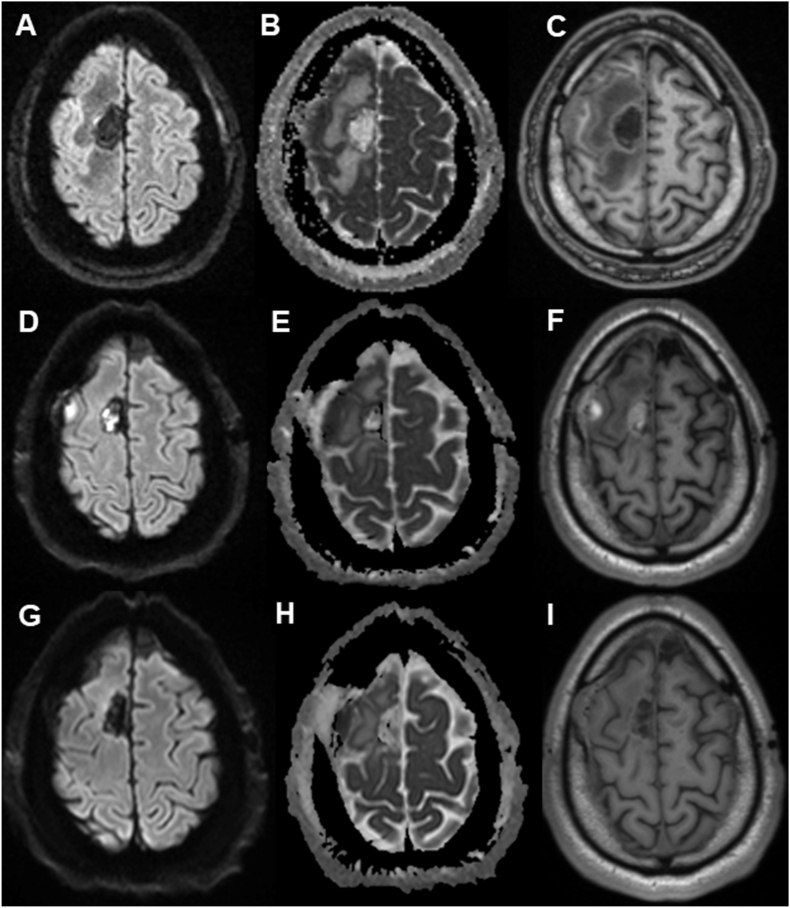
Temporal evolution of a postoperative brain abscess displayed on identical MRI slices using diffusion-weighted imaging (DWI), apparent diffusion coefficient (ADC) maps, and native T1-weighted imaging at different time points. (A–C) Immediate postoperative phase (≤7 days after first infection control surgery): (A) DWI, (B) ADC, (C) native T1-weighted image. (D–F) Early follow-up (4–12 weeks postoperatively): (D) DWI, (E) ADC, (F) native T1-weighted image. (G–I) Final follow-up examination: (G) DWI, (H) ADC, (I) native T1-weighted image.
